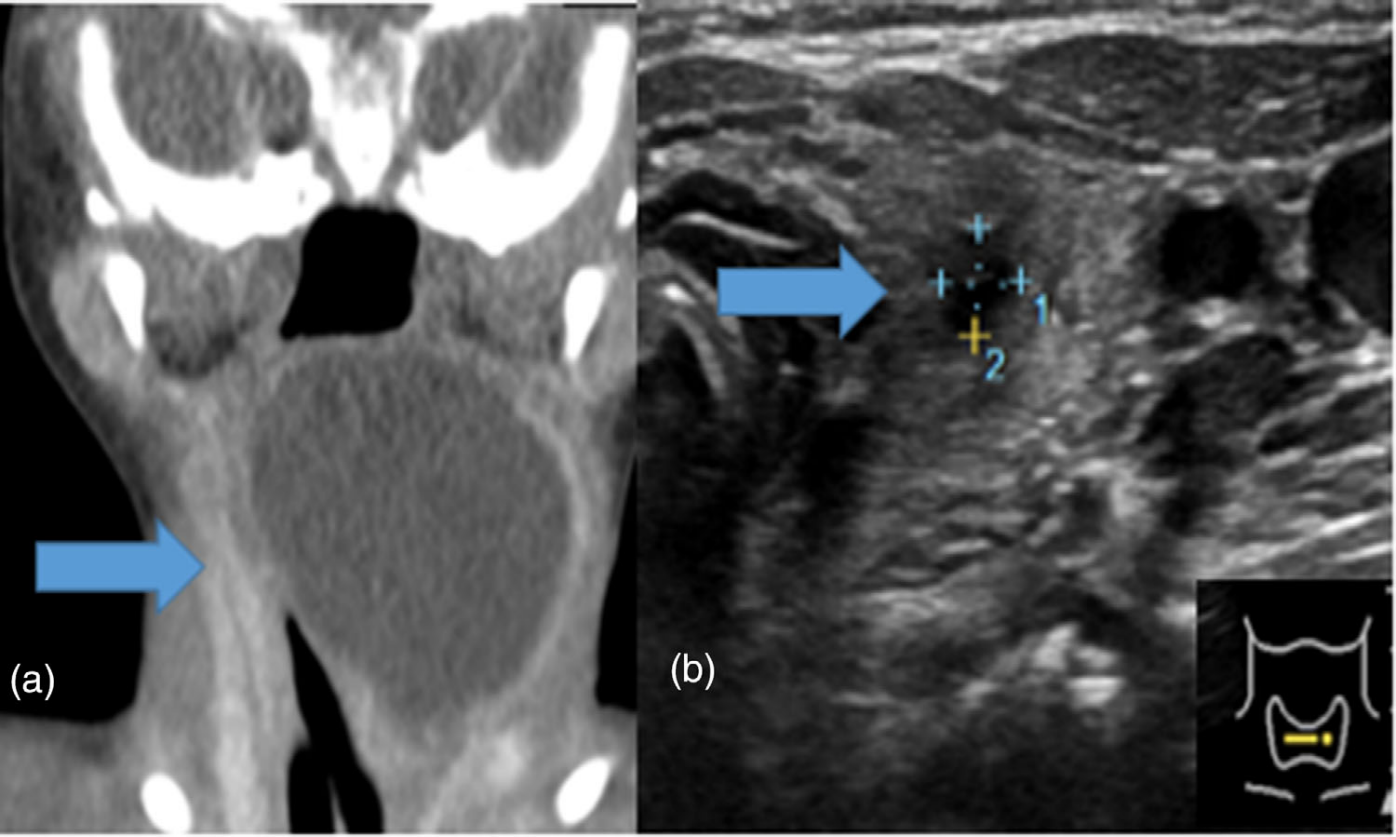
Computed tomography and ultrasonography findings of pyriform sinus cyst before and after ablation. Case 6 (a, b). (a) Computed tomography findings before ablation. A large cystic mass occupies the left side of the neck. (b) Ultrasonography findings one month after ablation. The cystic mass has remarkably shrunk
